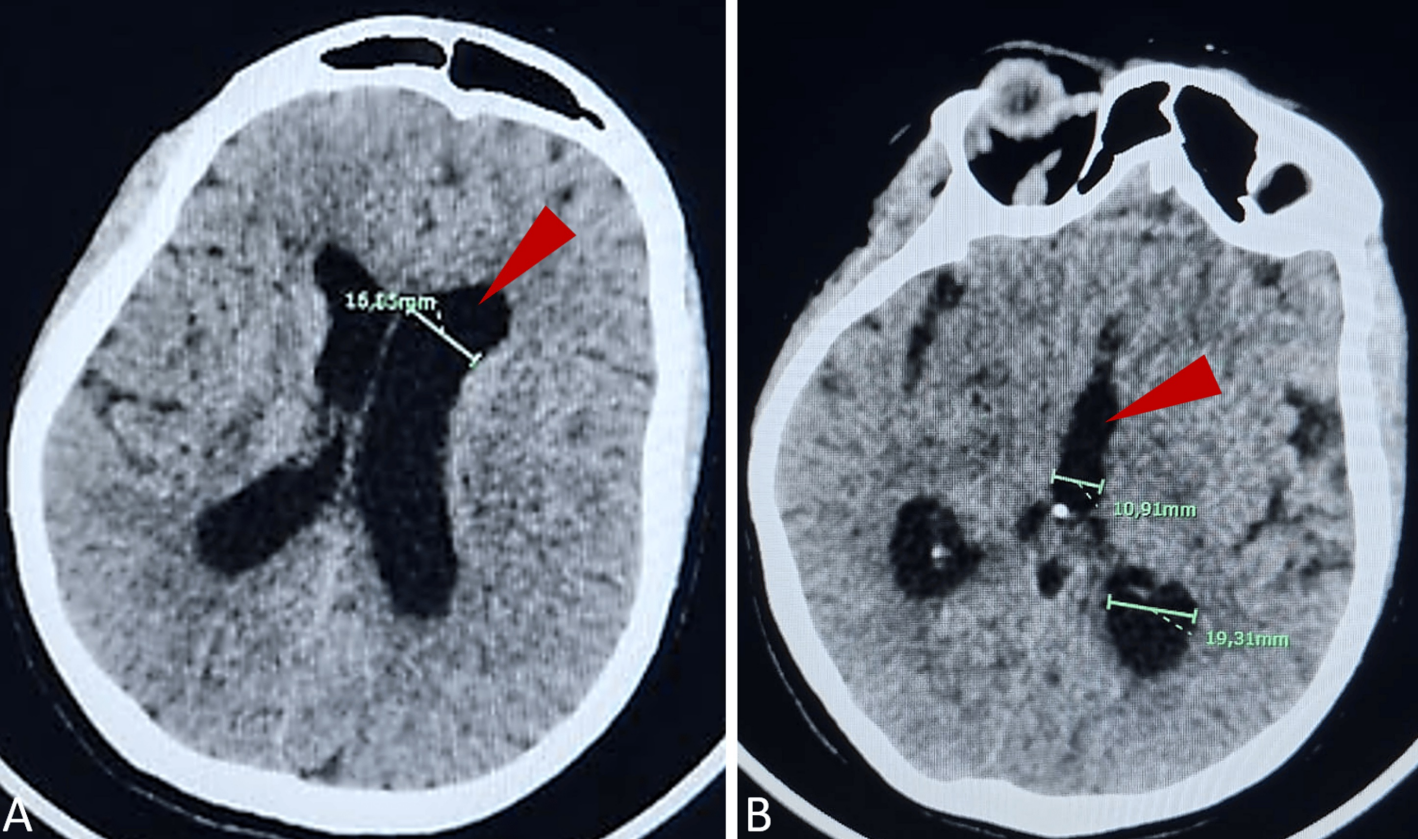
Transverse CT section of the brain showing hydrocephalus (arrow head) of the lateral ventricles (A) and the third ventricle (B).
